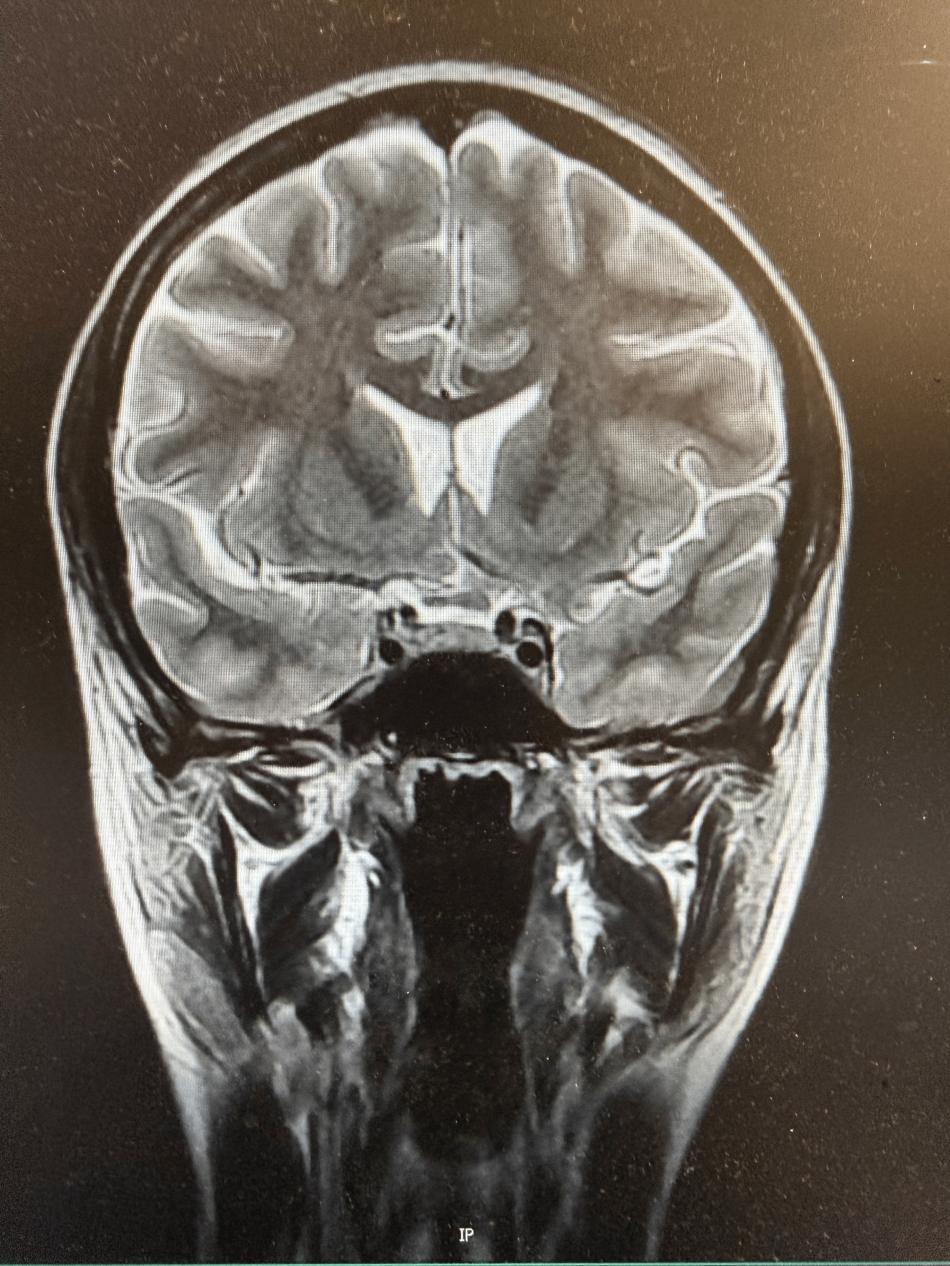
Coronal MRI through the sellar region Coronal T2-weighted MRI through the sellar and suprasellar region demonstrating normal pituitary morphology and intact optic chiasm. No sellar or suprasellar mass, haemorrhage or structural abnormality is identified.
